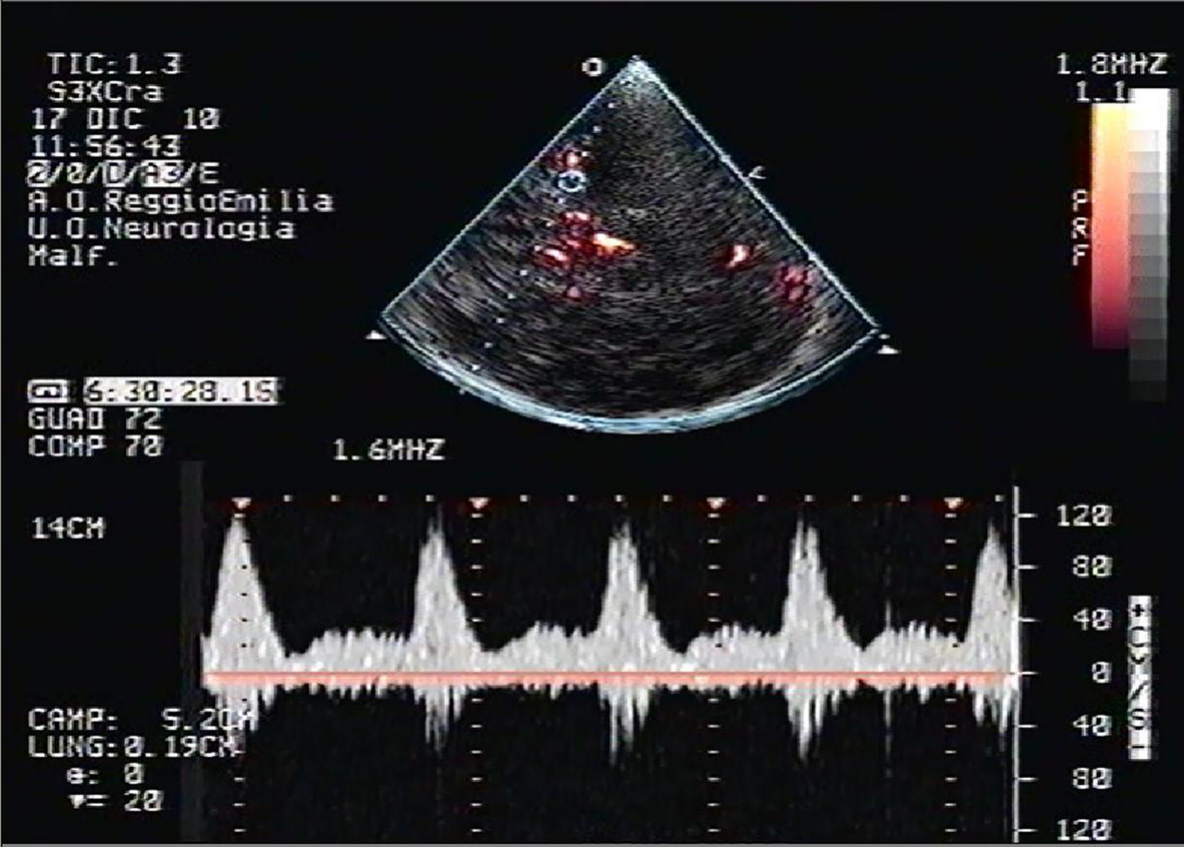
Transcranial Colour-Coded Duplex Sonography (TCCS) from the temporal bone window in axial scanning plane with Power-mode. The sampled right middle cerebral artery shows a continuous presence of multiple high-intensity transient signals (HITS), like a "curtain effect", 30 seconds after the release of the straining phase of the Valsalva manouver.
